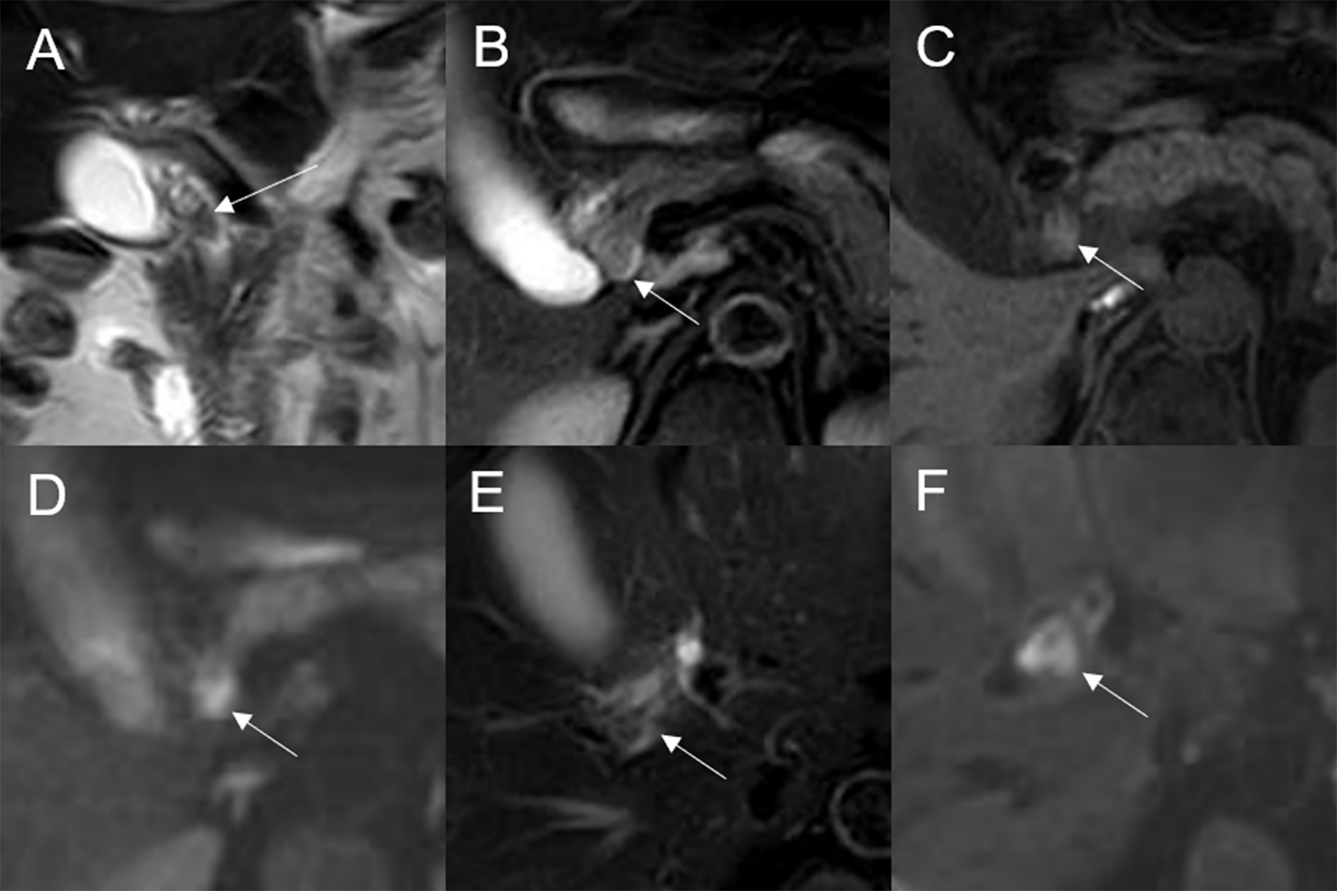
Malignant transformation of adenoma in the common bile duct. The eccentric mass with a frond-like superficial appearance (white arrow) shows hyperintensity on T2WI (A, B), isointensity on T1WI (C), and hyperintensity on DWI (D). The bile duct wall (white arrow) surrounding the lesion, without any abnormal signal on T2WI (E), shows hyperintensity on DWI (F).
